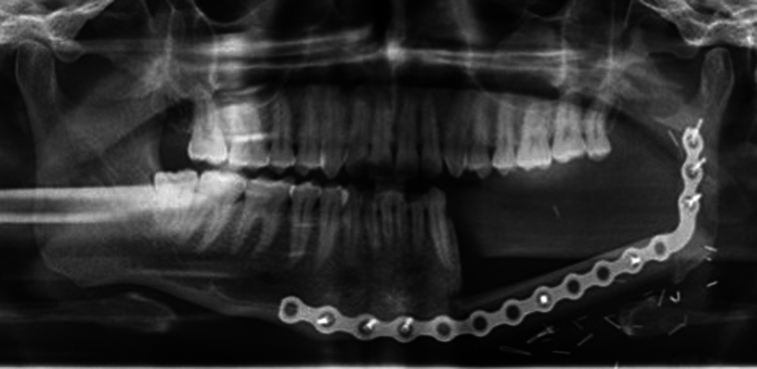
Panoramic radiograph after fibula reconstruction.
